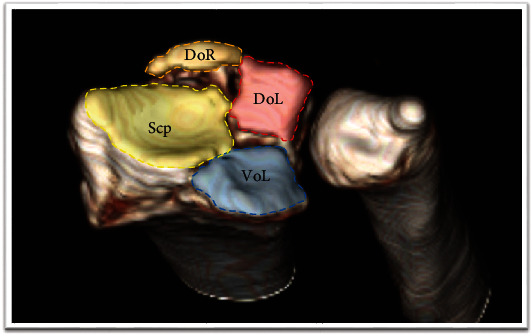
Initial CT scans. The articular surface was divided into six fragments: scaphoid facet (Scp), dorsal lunate facet (DoL), volar lunate facet (VoL), dorsal rim fragment (DoR), volar rim fragment, and central depression type. In this subject, the dorsal and volar lunate facet and dorsal rim fragment were involved.
